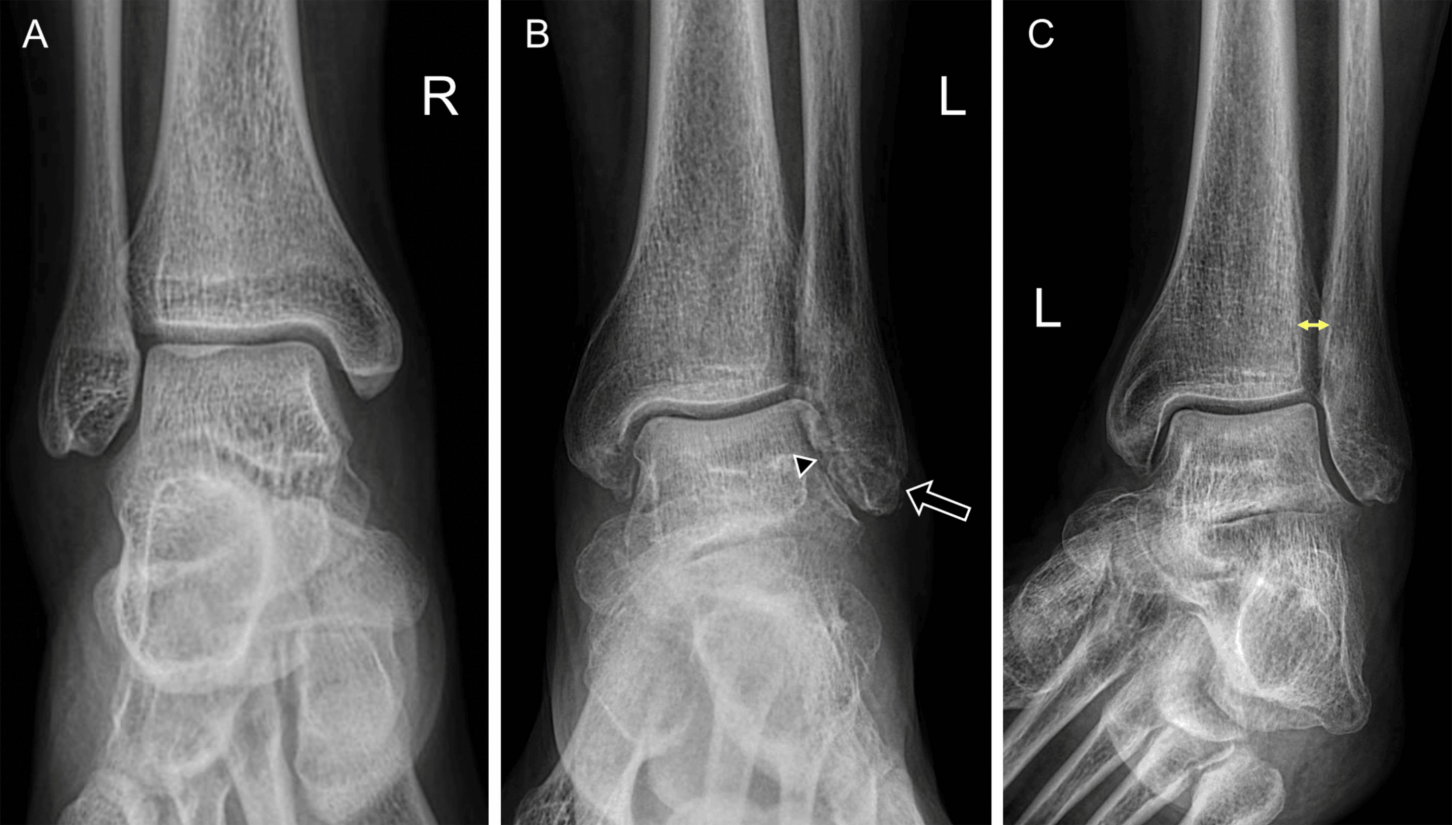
Radiographic appearance of syndesmotic widening. (A) Anteroposterior radiograph of a 19-year-old female demonstrates normal syndesmotic width; (B) anteroposterior radiograph of a 67-year-old female shows a distal fibula fracture (arrow) and decreased lateral joint space (arrowhead); (C) mortise projection of the same ankle shows a widened syndesmotic space (double arrow). Images belong to the authors; patient consent was obtained.
